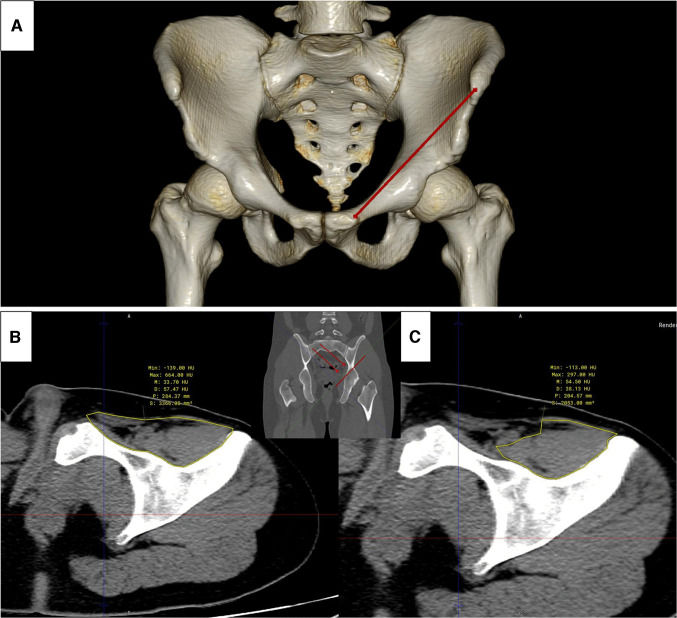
Inguinal ligament length measurement on 3D reconstructed CT scans (A). Using it as reference to align the planes, the cross-sectional area of the retroinguinal space (B) and of the lacuna musculorum (C) were measured on axial planes
